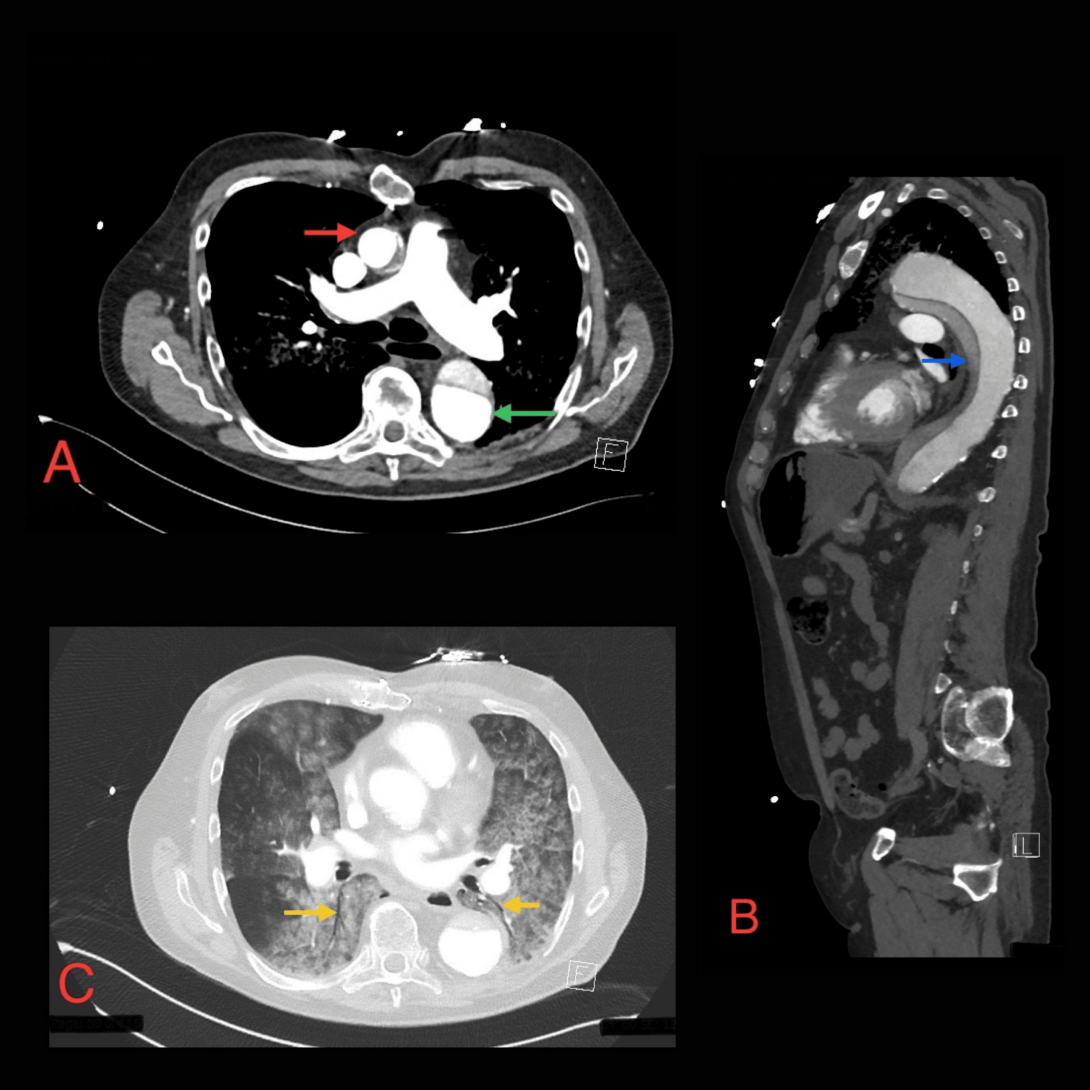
CT angiogram shows aortic dissection with bilateral pulmonary hemorrhage (A) An axial CT image shows aortic dissection affecting both the ascending (red arrow) and descending aorta (green arrow), classifying it as Stanford type A aortic dissection due to the involvement of the ascending aorta. Aortic dissection is associated with a high mortality rate. (B) Aortic dissection (blue arrow) on the sagittal CT image. (C) An axial CT image shows new extensive consolidation and ground glass opacity throughout both lungs with associated air bronchograms (yellow arrows), consistent with extensive pulmonary hemorrhage from acute aortic dissection. Pulmonary hemorrhage is a fatal complication of Stanford type A aortic dissection.
